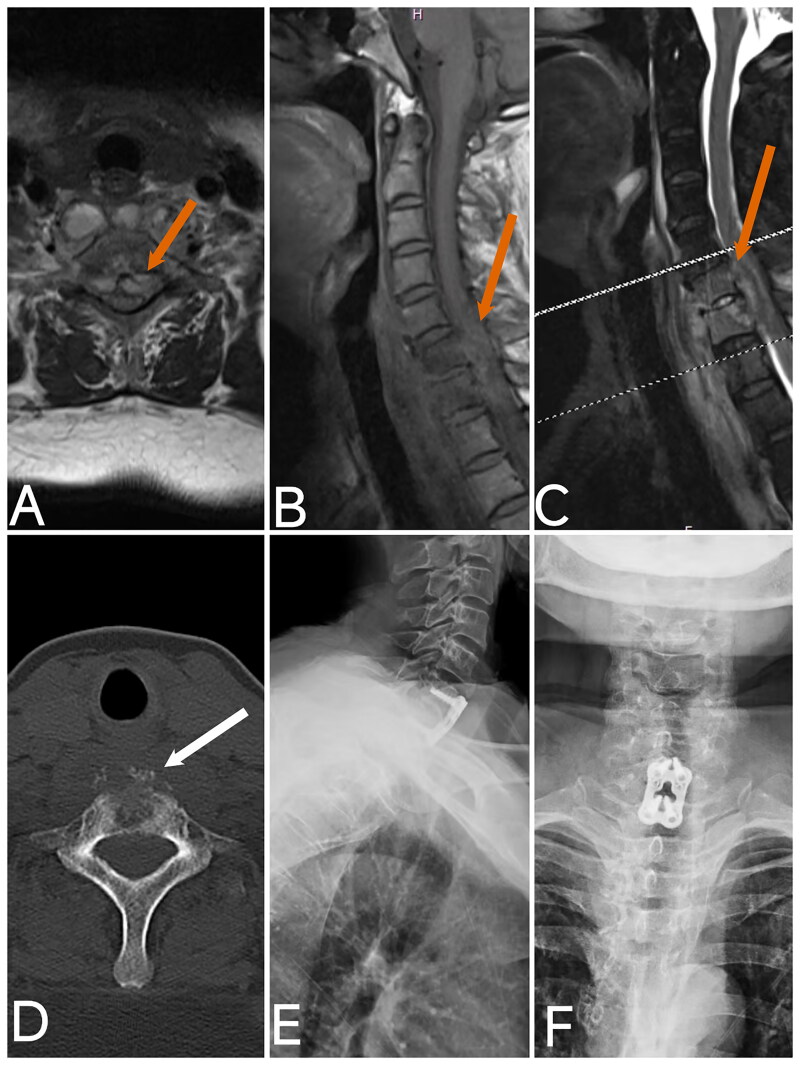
Image of case 3. A: Axial T2-weighted imaging (T2WI); B: T1-weighted imaging (T1WI); C: T2-weighted imaging with fat suppression (T2WI-FS) shows an epidural abscess with significant compression of the spinal cord (red arrow). D: CT reveals prevertebral bone destruction (white arrow). E, F: Postoperative X-ray at 3 months shows good positioning of the internal fixation.
